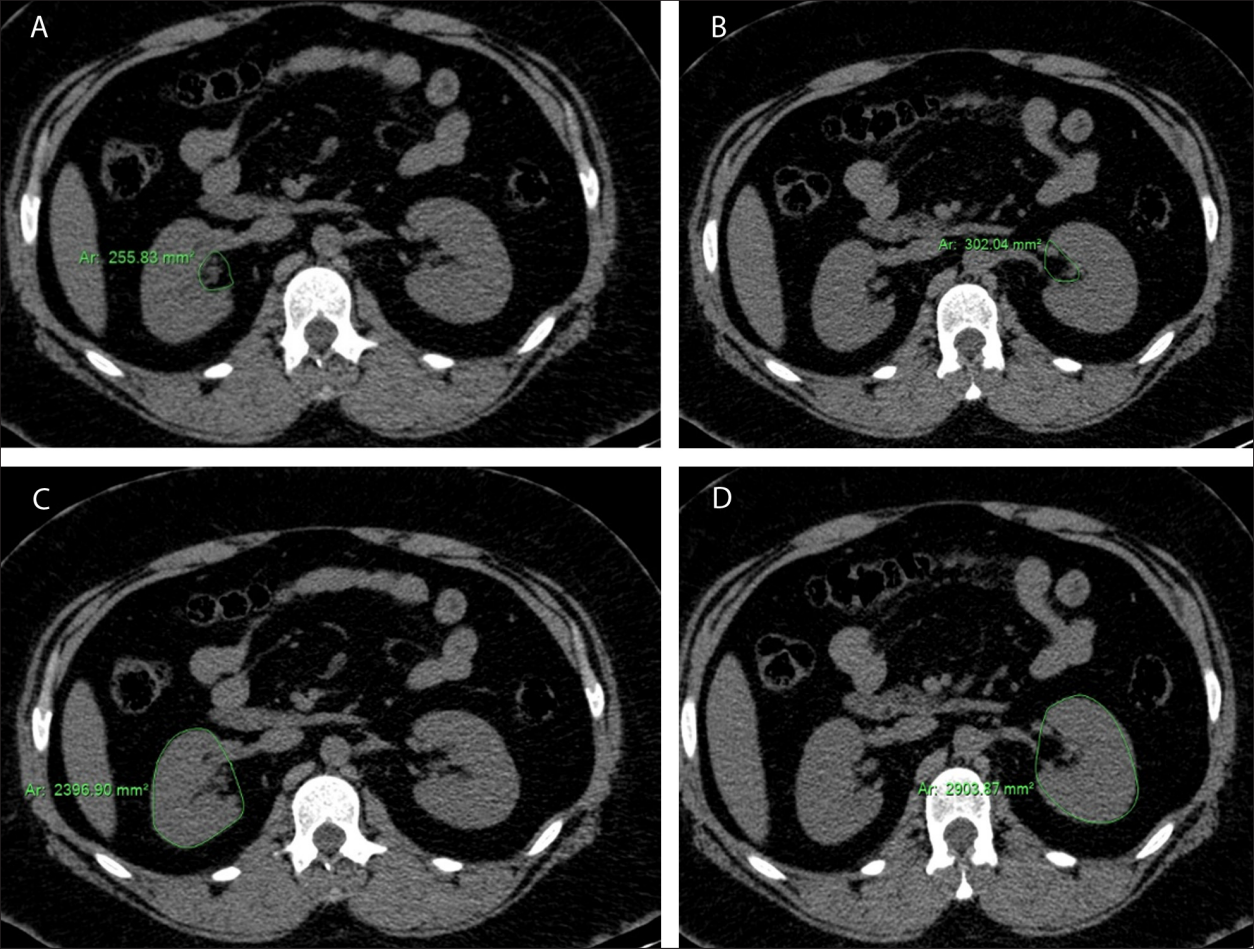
A control 57-year-old male patient, performed CT pelvi-abdominal examination with a history suggestive of appendicitis. A) and B) The RSFA on the right side was 255.83 mm^2^ and on the left side was 302.04 mm^2^. C) and D) The surface area of the right kidney and left kidney was 2396.9 and 2903.87 mm^2^ respectively.
